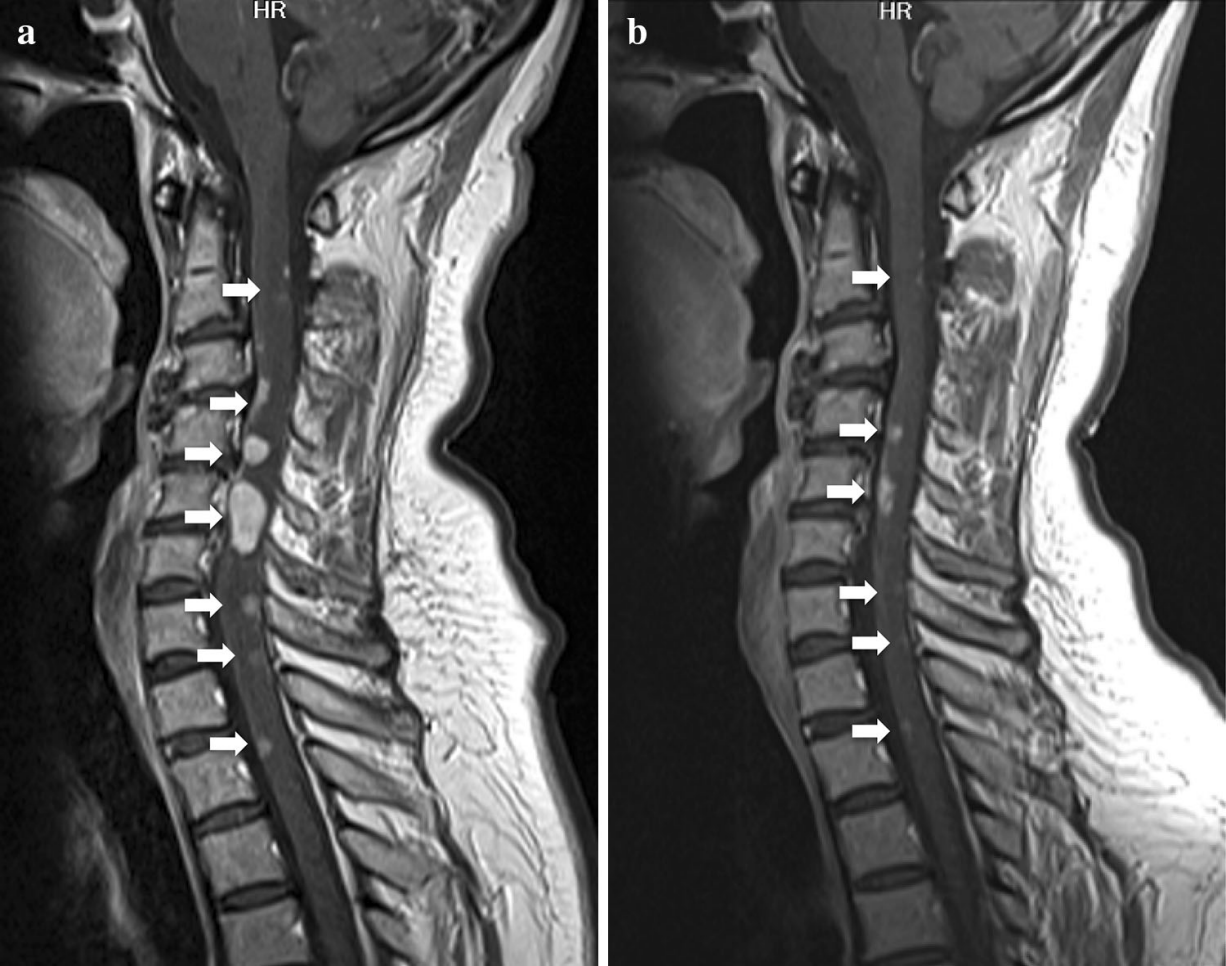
T1-weighted post-gadolinium MRI scans before a and after b 3 months of alectinib treatment revealed a marked response in multiple spinal cord metastases (see *arrows*)
